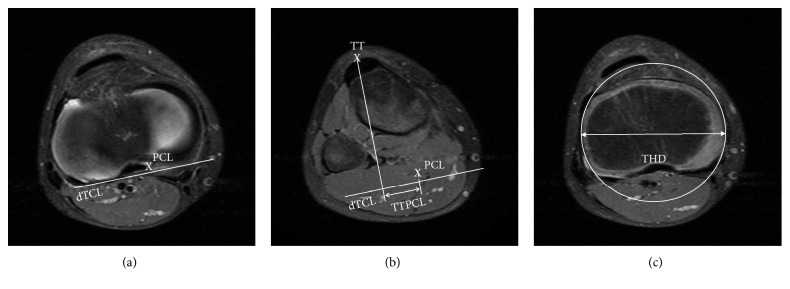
Measurement technique for the TTPCL/THD. Representative T2 MRI sequences acquired from a healthy 9-year-old boy. The mediolateral tibial tubercle-posterior cruciate ligament (TTPCL) distance was measured from (a) the medial border of the tibial insertion of the posterior cruciate ligament (PCL) and (b) the midpoint of the tibial tubercle (TT). The distance was measured parallel to the dorsal tibial condylar line (dTCL). The bony margins in the MRI scans were utilized except when measuring the tibial head diameter (THD).To determine the THD, the transversal plane with the maximum diameter of the tibial head was identified by the examiner (c).
